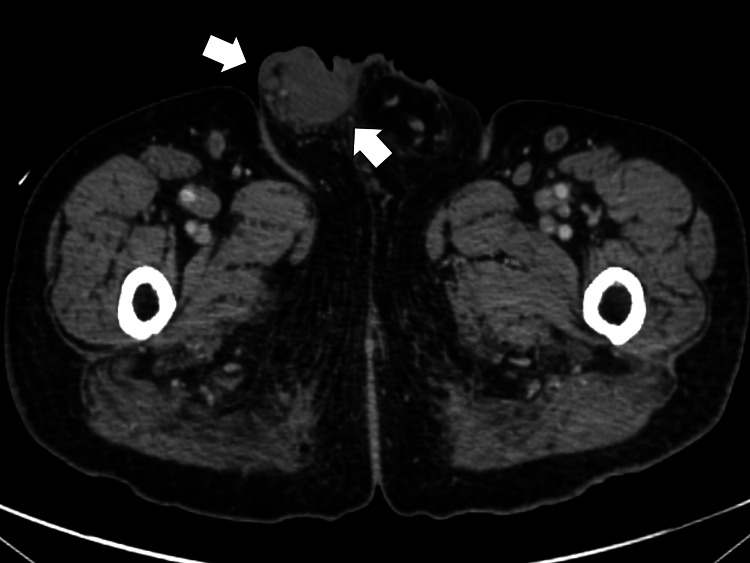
Pelvic computed tomography showing right testicular enlargement with edema (white arrows)
